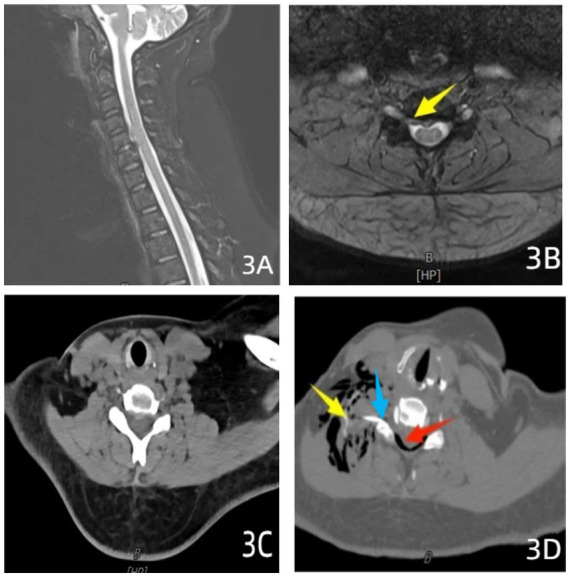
CT-guided transforaminal injection in a 53-year-old male patient. (A) Sagittal T2-weighted fat-suppressed MR image demonstrating C5/6 disc herniation compressing the anterior margin of the dural sac. (B) Axial T2-weighted fat-suppressed MR image revealing right-sided foraminal-type disc herniation at C5/6 (yellow arrow). (C) Axial CT scan confirming disc herniation without calcification. (D) CT-guided right transforaminal injection of ozone combined with anti-inflammatory solution, with red arrows indicating ozone distribution posterior to the dural sac and within soft tissue spaces, and yellow arrow indicating contrast medium extravasation. The blue arrow indicates that the tip of the needle is located at the anterior border of the facet joint.
